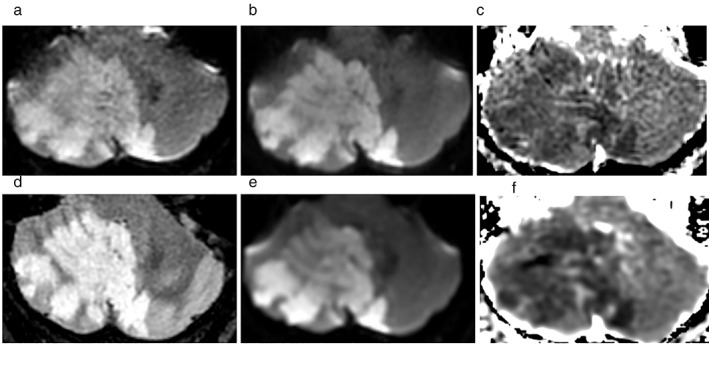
Large ischemic lesion; 49‐year old male with recurrent episodes of vertigo for 4 days and a large acute ischemic lesion in the right cerebellar hemisphere extending into the left hemisphere visible as a hyperintense lesion on EPIMix T2‐FLAIR (a) and EPIMix DWI (b) and as a hypointense lesion on EPIMix ADC (c). The acute infarction is just as visible on the corresponding sequences of routine clinical MRI (d–f).
